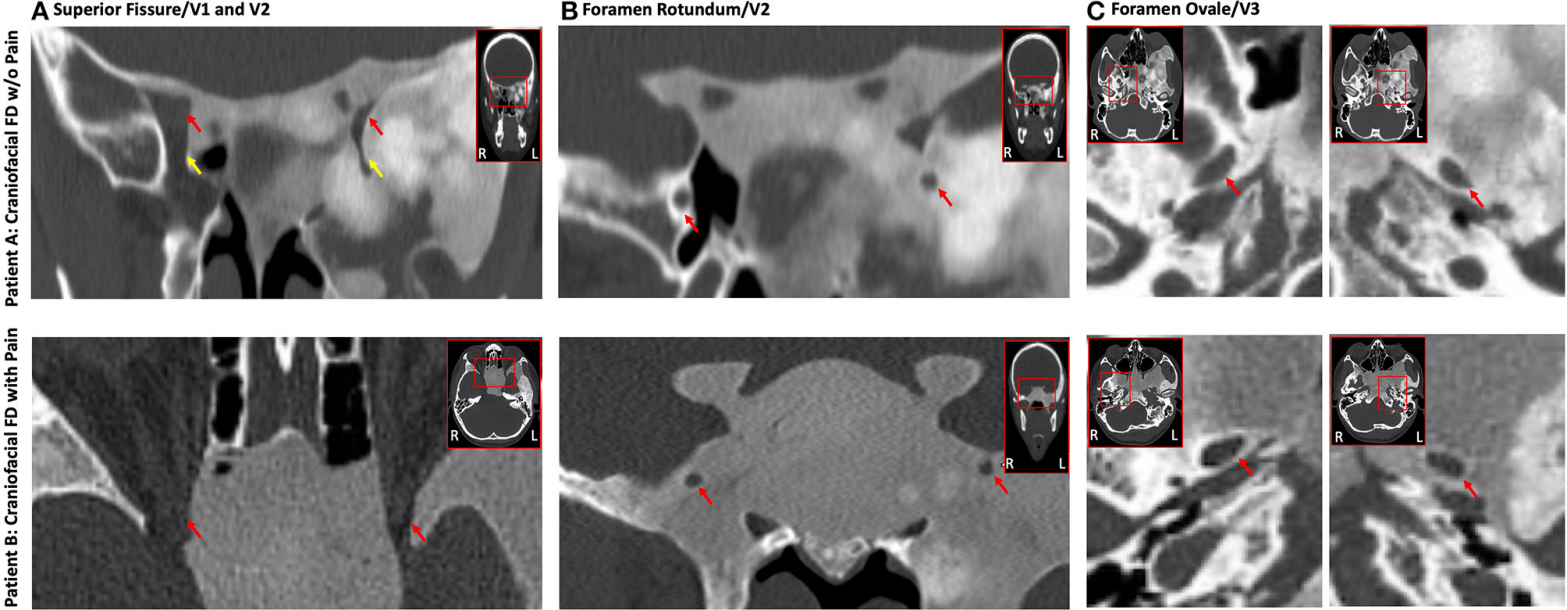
Impact of FD on the trigeminal nerve system. Craniofacial FD lesions caused a highly similar impact on conduits carrying trigeminal nerves in both Patients A and B. Displacement and narrowing of the V1 (A), V2 (B), and V3 (C) divisions of the trigeminal nerves was evident on CT for both FD patients with generally a greater impact on left hemisphere structures relative to the right. The top (Patient A) and bottom (Patient B) rows show coronal or axial cross-sections from CT. (A) Constriction of the superior orbital fissure (red arrow) and inferior orbital fissure (yellow arrow; Patient A only). (B) Narrowing and displacement of the left foramen rotundum (red arrows). (C) Narrowing and displacement of the left foramen ovale (red arrows). (C) All data were read by a board-certified neuroradiologist (Dr. Romo).
